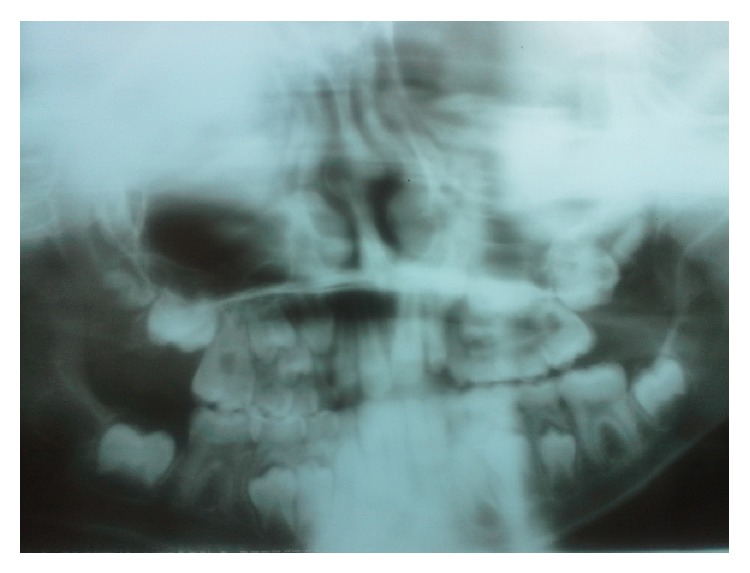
Radiographic examination.
